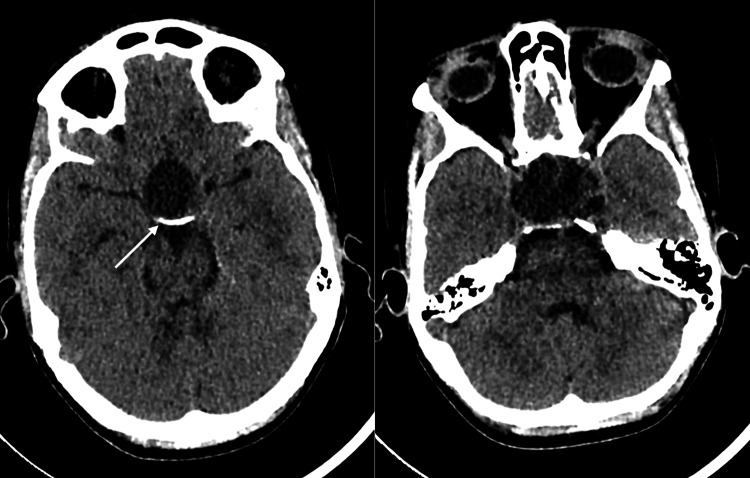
Axial non-contrast computed tomography of the head. Large cystic sellar/suprasellar mass with peripheral calcifications (arrow) and causing excessive expansion of the sella.
